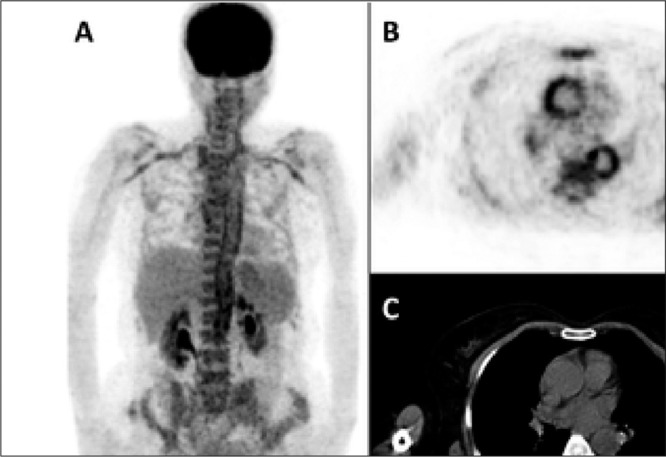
PET images of anterior MIP (A), transaxial PET (B) and CT (C)show involvement in large vessels (aorta, subclavian arteries, andcarotid arteries). A biopsy of temporal artery revealed giant cell arteritis(temporal arteritis)
